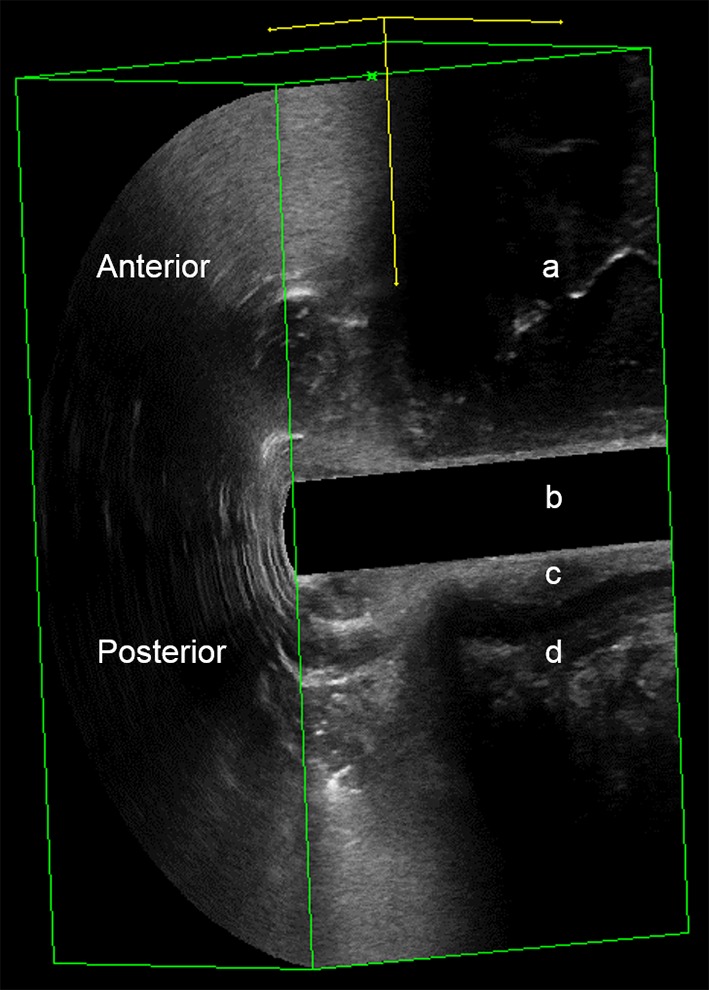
Endoanal ultrasound of low rectovaginal fistula (RVF): *a* RVF tract; *b* ultrasound probe; *c* internal anal sphincter; *d* external anal sphincter
